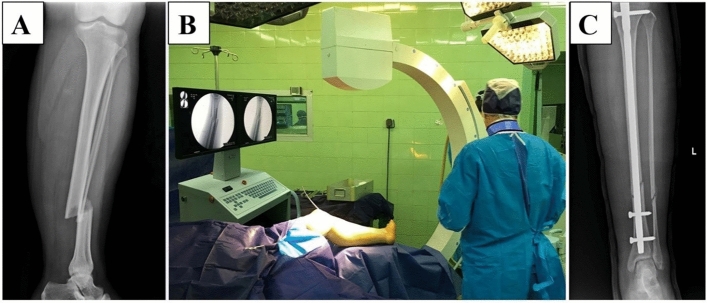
Interlocking of the intramedullary nail using FH technique, (A) The X-ray image of the patient's left foot before the surgery, (B) Inserted guide-wire inside the tibia bone under fluoroscopy control, (C) The X-ray image of the patient's left foot one day after the surgery.
